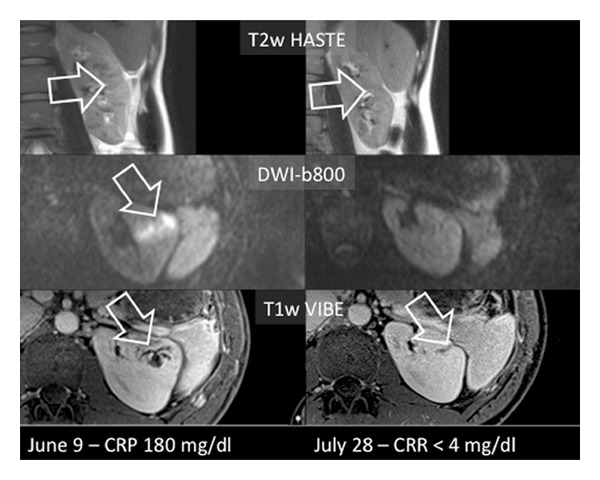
Pyelonephritis in a 31-year-old female patient of the left kidney who was imaged initially to assess the extent of renal involvement for possible operation and reimaged after 7 weeks of antibiotic therapy. Left column: a clear infectious focus can be easily seen on the DWI images and on the venous T1w images and the signal alterations of the kidney appear diffuse on T2w imaging. Right column: after successful antibiotic therapy with normal CRP value, no pathologic signal changes can be seen on the DWI images. The T1w and T2w images show moderate scarring of the formerly affected parenchyma (arrows).
